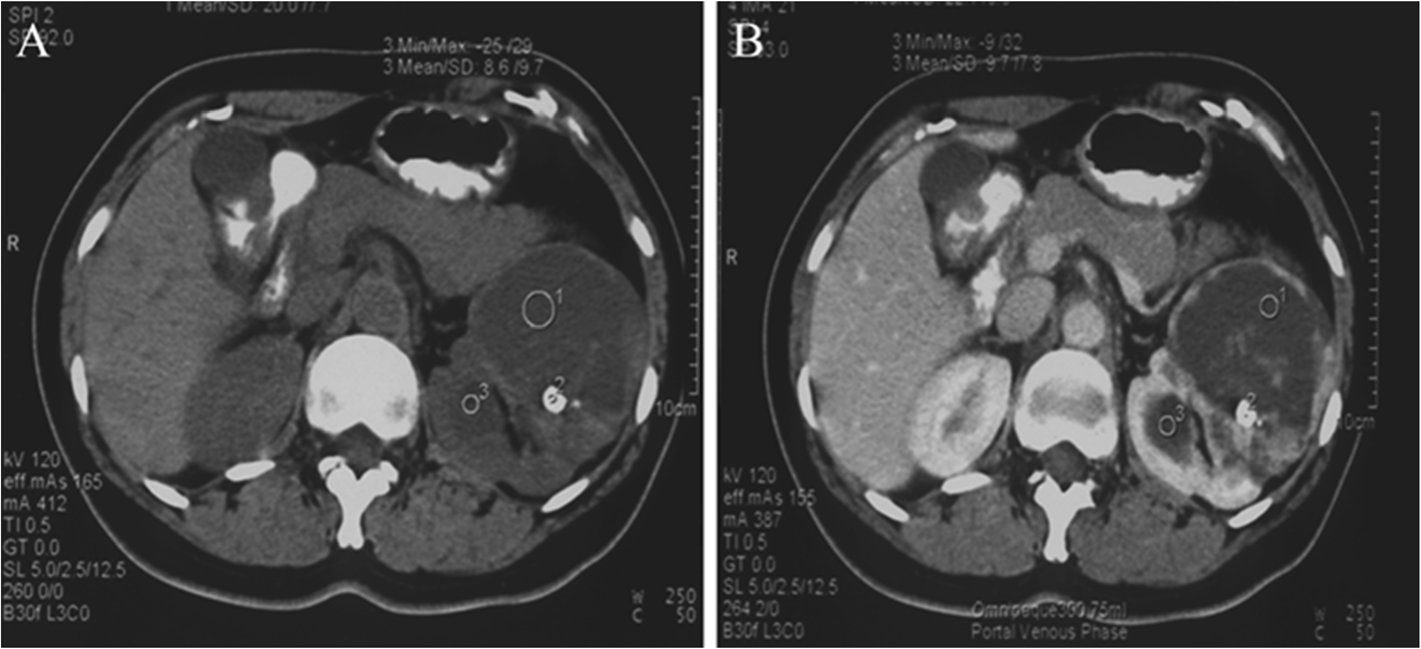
A typical CT scan image of a left kidney with CRCC. (A)The CT scan showed the thick and irregular capsule walls surrounding several cysts with hyperdense septa and nodules. (B)The enhanced CT scan image showed the intense enhancements and calcification of capsule walls, septa, and nodules. The debris, floc, and blood clots were also visible in the hydatid fluid.
